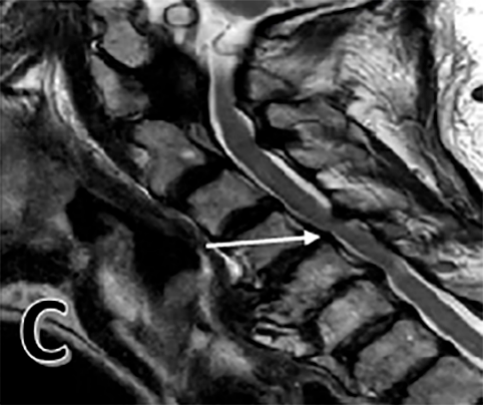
Pre-operative T2 sagittal MRI showing spondylosis and central stenosis from C4-6 (white arrow).
